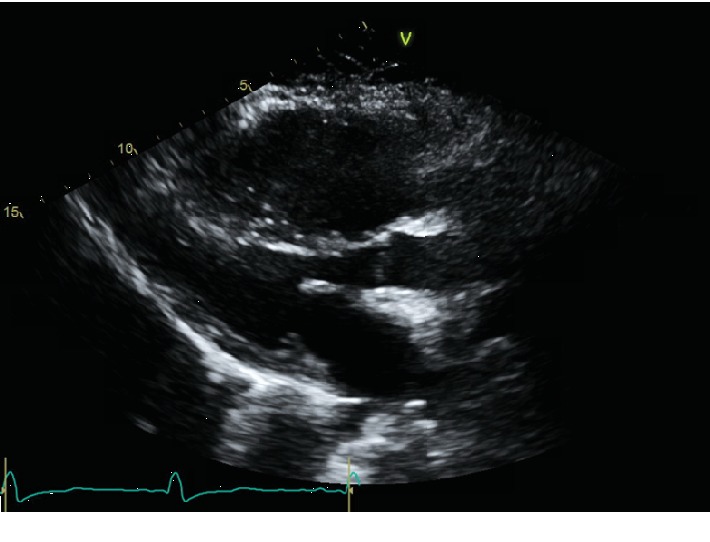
Transthoracic Echocardiogram Parasternal Long Axis View showing enlarged right ventricle from volume overload.
